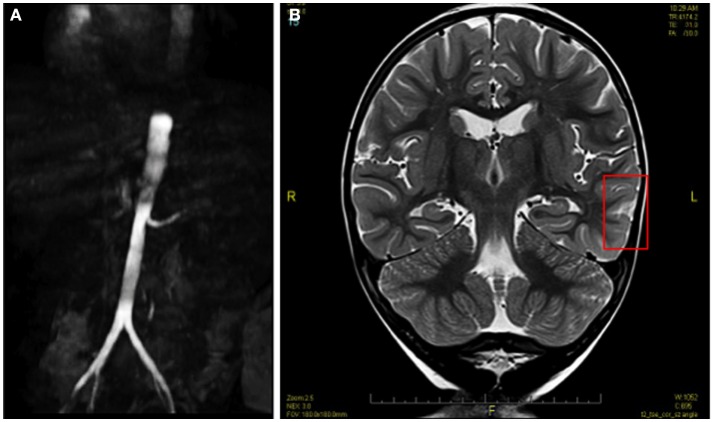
Pre-angioplasty MRI image showing high-grade proximal renal artery stenosis (A) and hyperintense lesions of PRES (B).
